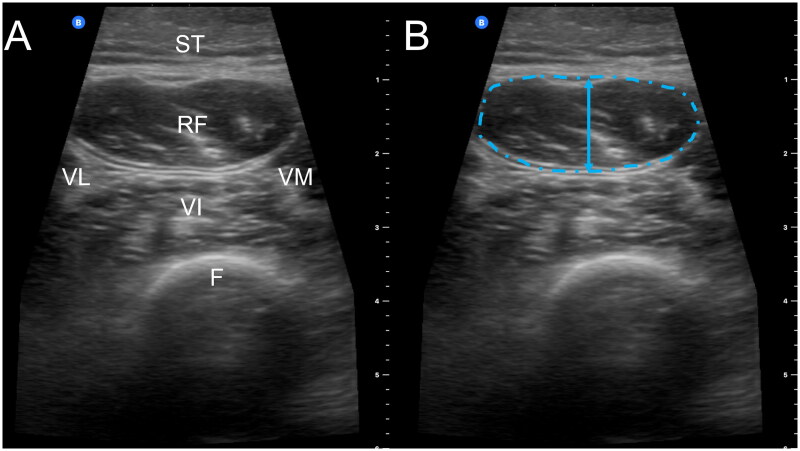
(A) Ultrasound image of the quadriceps acquired at two-thirds the distance from the anterior superior iliac spine to the superior aspect of the patella with 6 cm of depth demonstrating subcutaneous tissue (ST), rectus femoris (RF), vastus lateralis (VL), vastus medialis (VM), vastus intermedius (VI), and femur (F). (B) The blue arrow denotes rectus femoris muscle thickness while the blue dashed outline delineates the rectus femoris cross-sectional area. Rectus femoris echointensity is calculated from the pixel density within the rectus femoris cross-sectional area.
